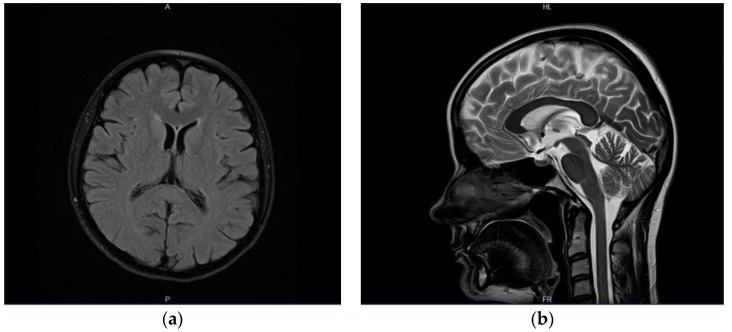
Another follow-up MR examination after 13 months. Substantial regression of the SCC lesion; discrete, indistinct hyperintensity on axial T2w-TIRM (dark fluid) images (a). No signal abnormalities were visible within splenium on other MR sequences—as visible on conventional T2-weighted (blade) sagittal image (b).
